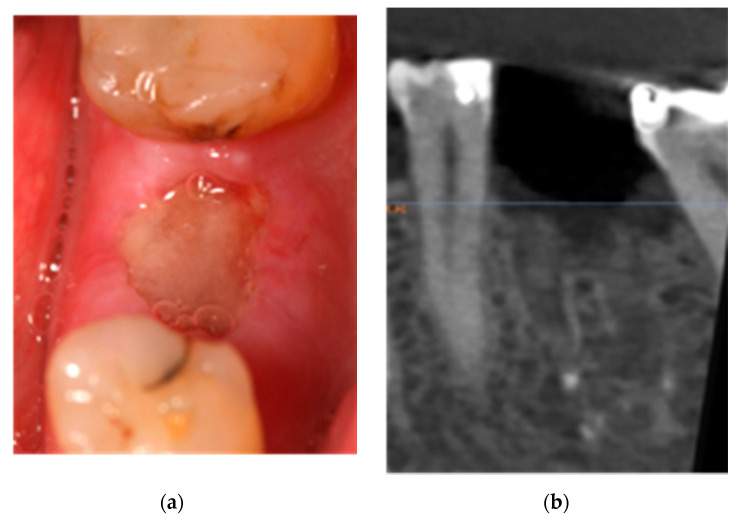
(a) Clinical aspect of the injury. (b) X-ray of the affected area.
